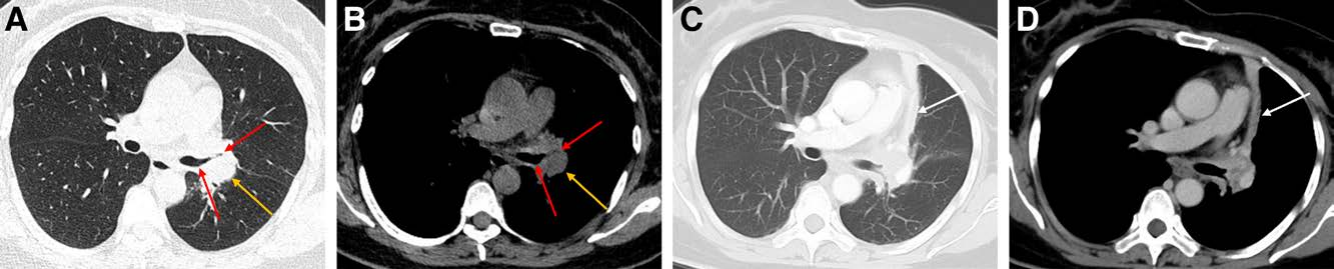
Pretreatment chest CT findings in patient. A mass in the left hilum (yellow arrows) and luminal narrowing of the left main bronchus and left upper/lower lobe bronchi (red arrows). Strip-like consolidative atelectasis in the lingular segment of the left upper lobe (white arrows). CT = computed tomograph.
